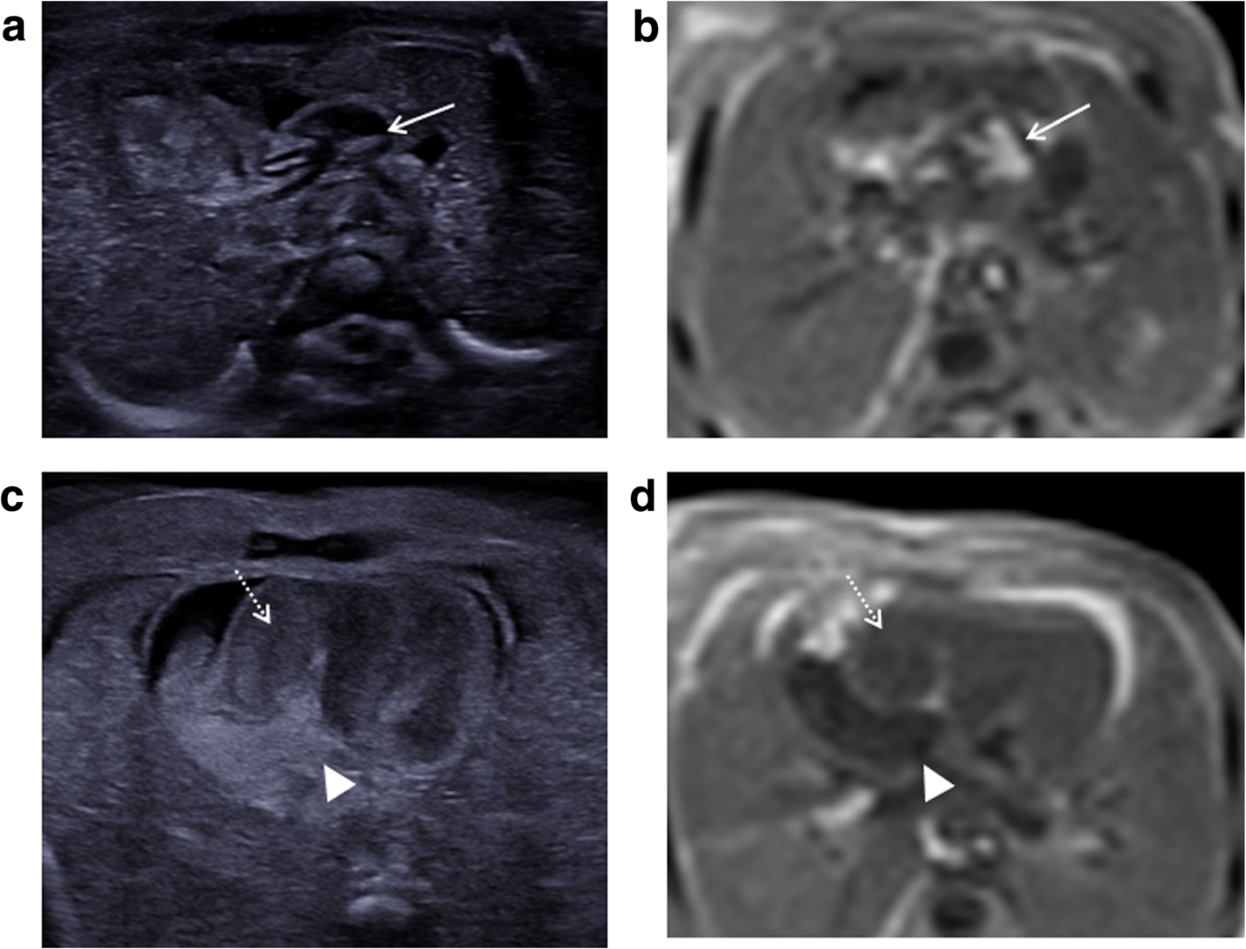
Supravalvular pulmonary artery stenosis in a foetus at 21 weeks gestation. The imaging was performed 5 days after termination of pregnancy. Transverse images of the outflow tracts on post-mortem ultrasound (a) and T2 weighted MRI at 1.5T (b) both demonstrate a dilated main pulmonary artery (solid arrows). The transverse four cardiac chamber view on ultrasound (c) and MRI (d) show a dilated and thick-walled right ventricle (dotted arrow) and small atrial septal defect (arrowhead). Given the small size of the foetus, the findings were more easily appreciated on ultrasound than MRI
